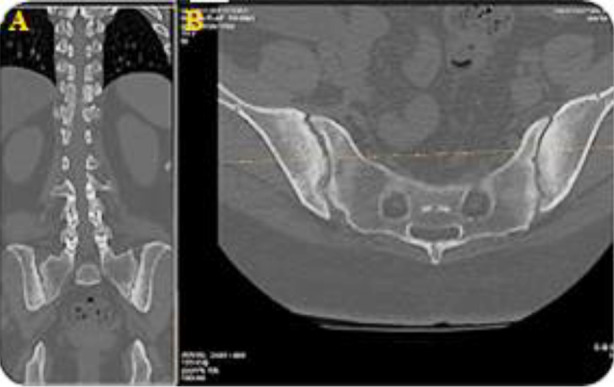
CT scan image depicting sacroiliitis in a patient with ankylosing spondylitis. A; coronal plane, B; Axial plane.
